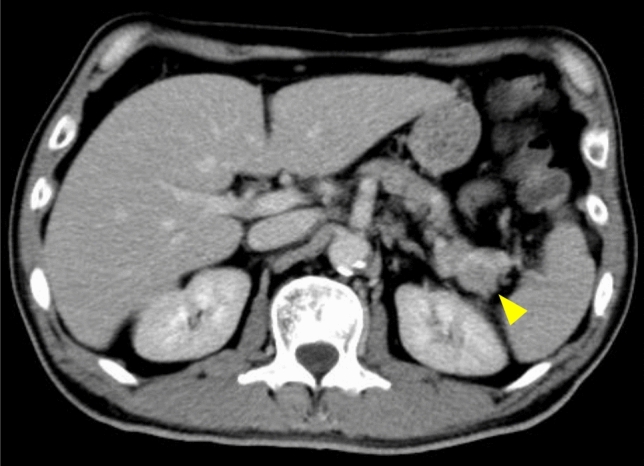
Contrast-enhanced computed tomography of the chest and abdomen performed at the referring hospital revealed a 10 mm hypovascular mass in the pancreatic tail (yellow arrowhead). No evidence of distant metastases, including nodular bone metastases, or other findings suggestive of malignancy was observed
